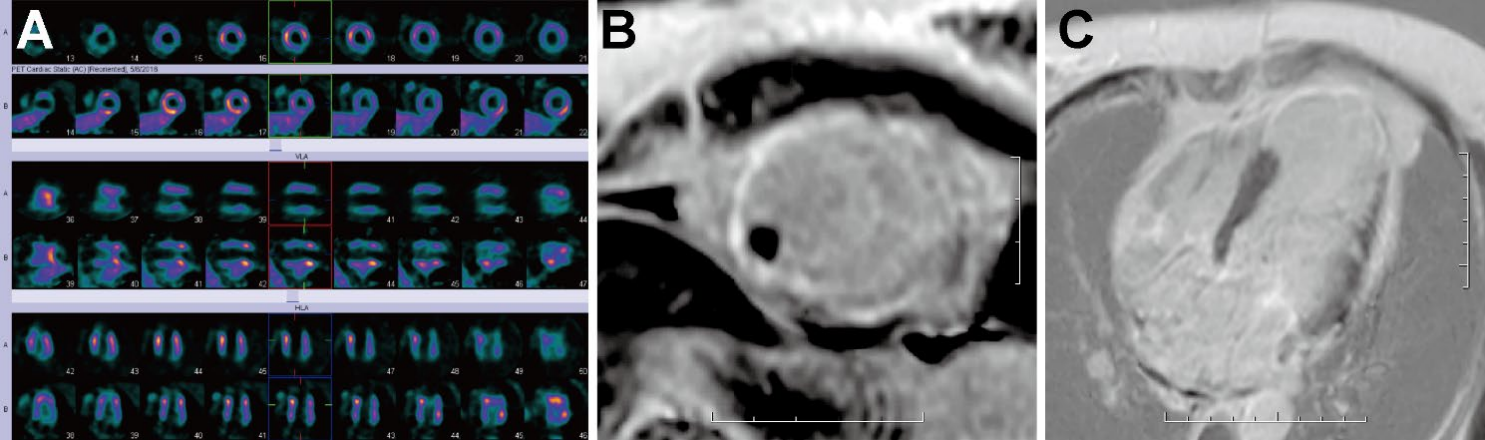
(A). Rest ^99m^Tc-MIBI myocardial SPECT images showed myocardial perfusion defects in the apex and inferior basal segment of the left ventricle. 18 F-FDG myocardial metabolism images revealed metabolic defects. (B), (C). The ventricular aneurysm accounted for about one-quarter of the left ventricular cavity. First-pass perfusion imaging demonstrated perfusion defect in the above segments. An intracardiac mural thrombus was observed
